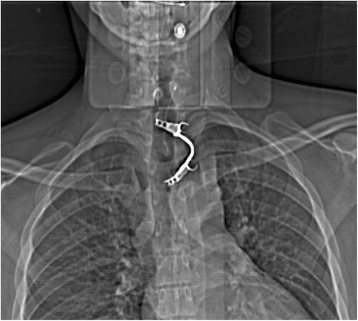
Radiograph showing the ingested denture in the proximal esophagus
